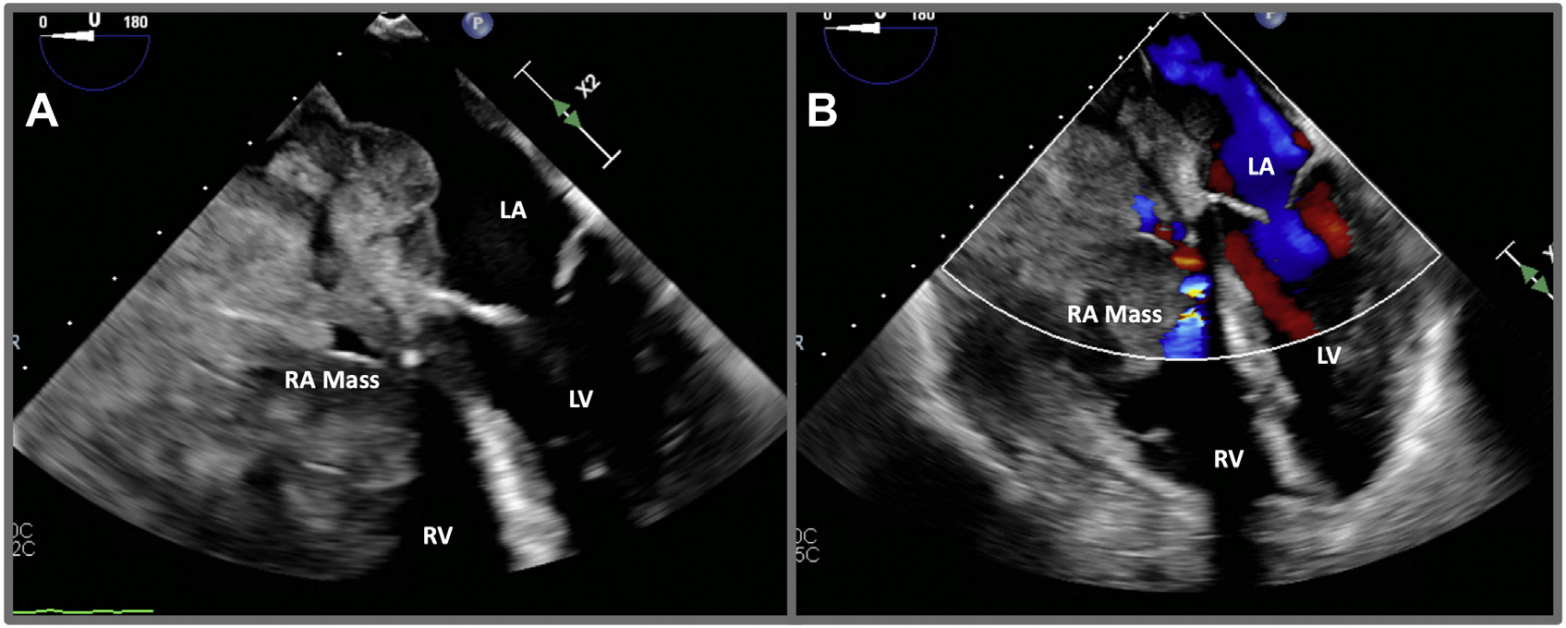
Mass in the RA Seen on TEE (A) Transesophageal echocardiography (TEE) imaging displaying a large mass in the right atrium (RA) that was protruding into the right ventricle (RV), as well as across the interatrial septum into the left atrium (LA), indicating a malignant process. (B) Accelerated color flow Doppler imaging indicating flow restriction across the tricuspid valve (TV), likely in the location where balloon intervention was performed. LV = left ventricle.
